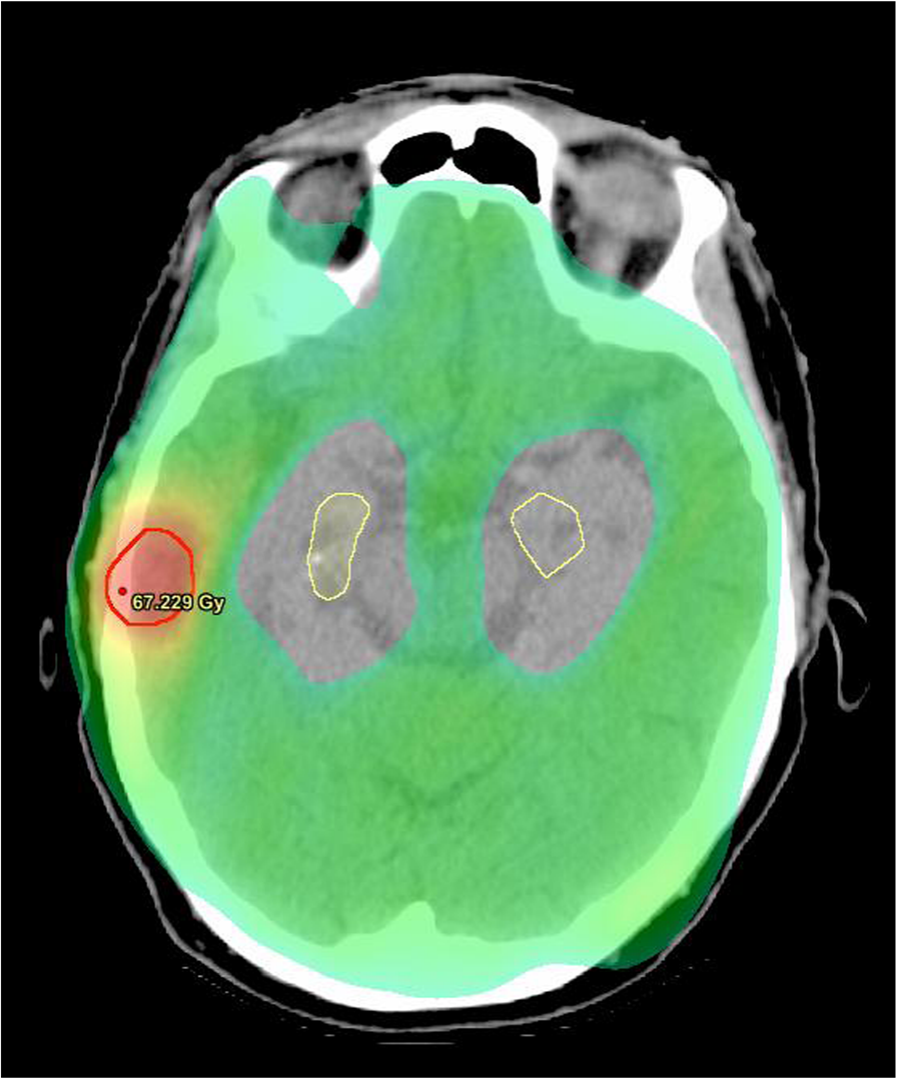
VMAT dose colour wash for WB_ SIB_HA on planning CT of a representative patient. Dose to metastasis was escalated (red, 63 Gy), WB at lower dose (green, 32.5 Gy), with HA (contour yellow).
